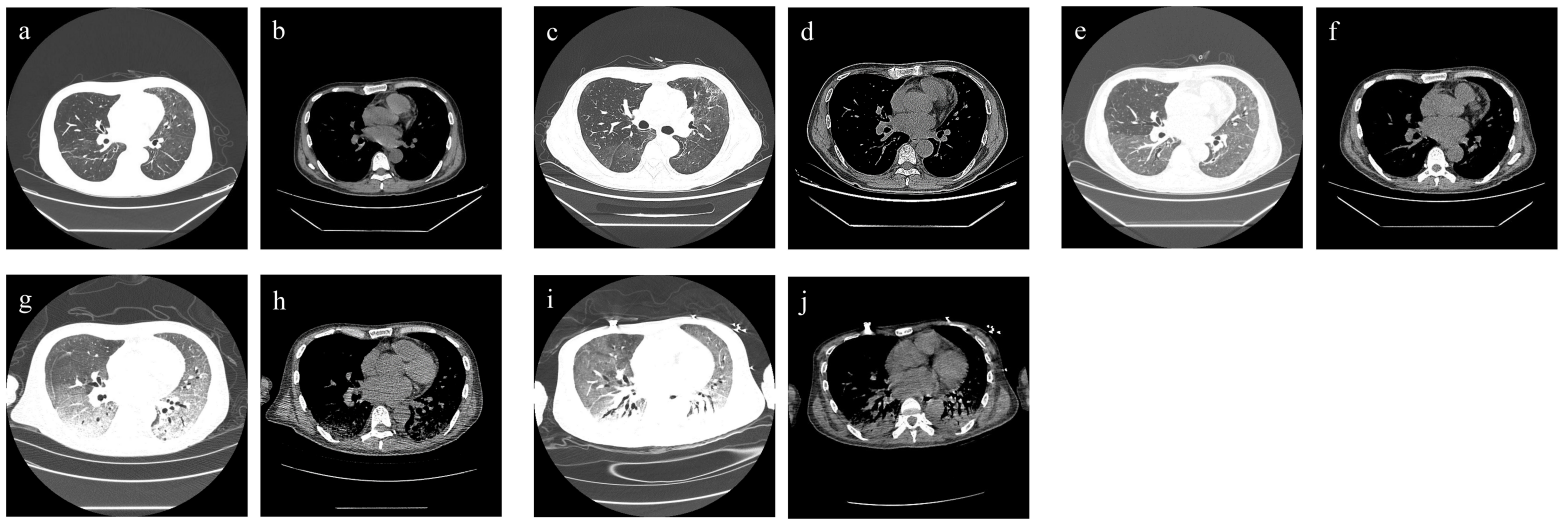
Chest CT findings of case 2. (A, B) For admission performance: multiple cords and patchy shadows in both lungs. (C, D) For the third-day performance: double lung (see multiple cords), patchy shadow, part of the range is larger than before, and the density is higher than before. (E, F) For the 10th-day performance: bilateral lung texture enhancement, bilateral lung parenchyma diffuse mist shadow, bilateral lung (see multiple flocculents), and cord-like density increased shadow. (G, H) For the 21st-day performance: bilateral lungs (see multiple large patchy density increased shadow) and fuzzy boundary. (I, J) For the 36th-day performance: decreased brightness of both lungs, bilateral lungs (see multiple large patchy increased density shadow), and blurred boundaries (see air bronchogram).
